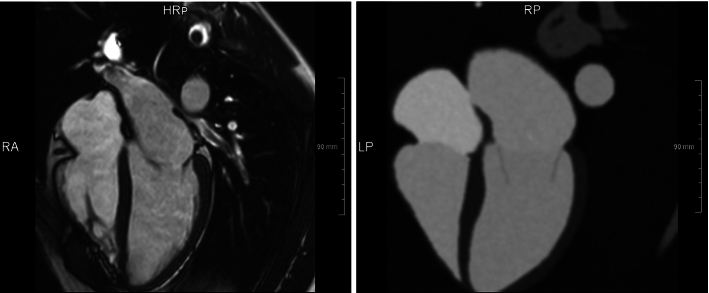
Real vs synthetic images comparison. MRI acquired on healthy volunteer (left) with similar parameters to our rLAX18 generated synthetic image based on the anatomy derived from CT and intensities from CMR (right)
